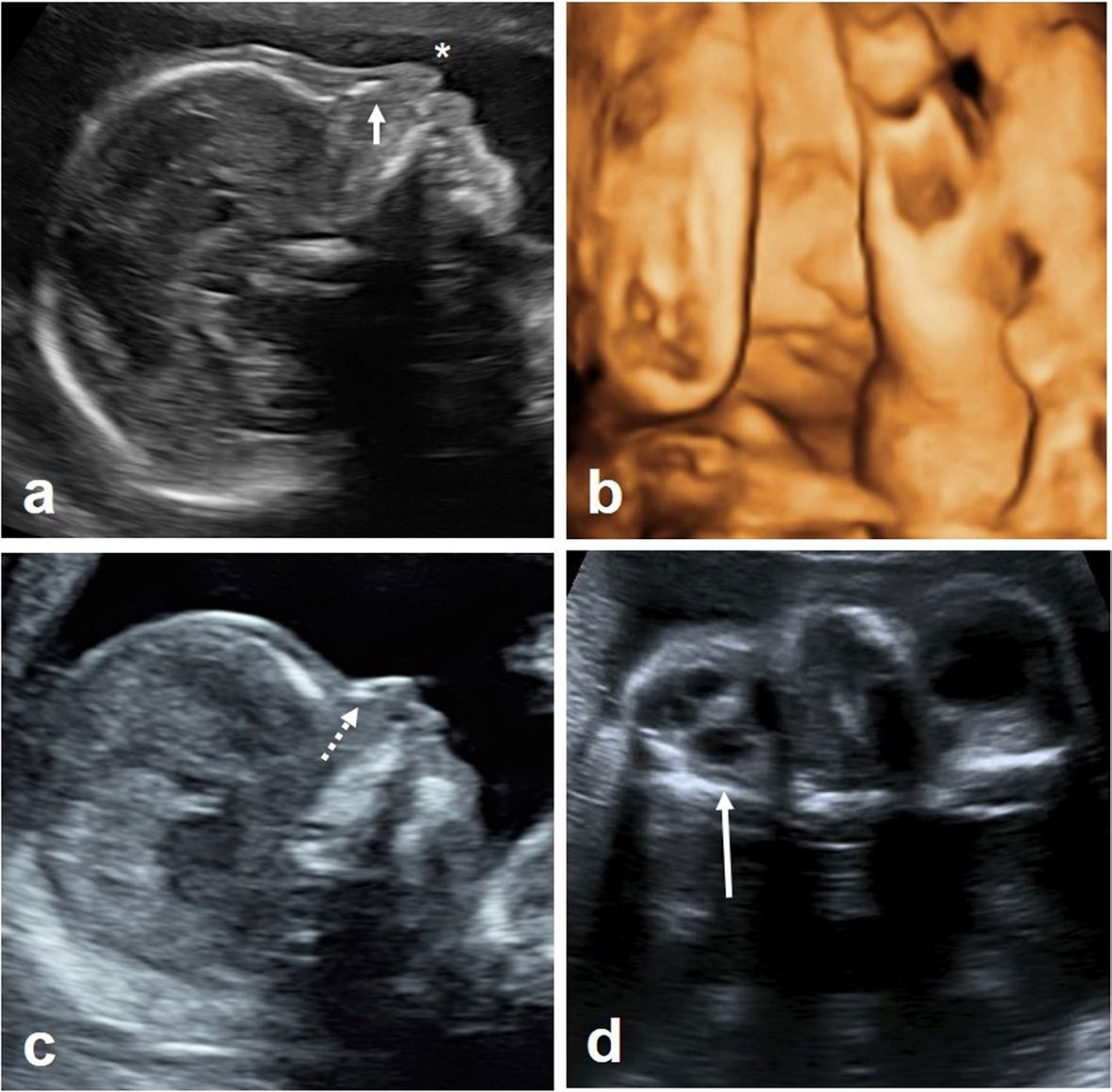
Prenatal ultrasound in fetuses with Wolf-Hirschhorn syndrome: a + b) show 2^nd^ trimester 2D- and 3D-ultrasound of the fetal profile, note the hypoplastic nasal bone (short, white arrow) as well as the beaked and triangular shape of the tip of the nose; c) shows 1^st^ trimester ultrasound of the fetal profile at 14 + 3 weeks ‘; note the hypoplastic nasal bone (dotted arrow); d) shows persistent hyperplastic primary vitreous (long, white arrow) in a fetus with WHS at 23 + 0 weeks‘
